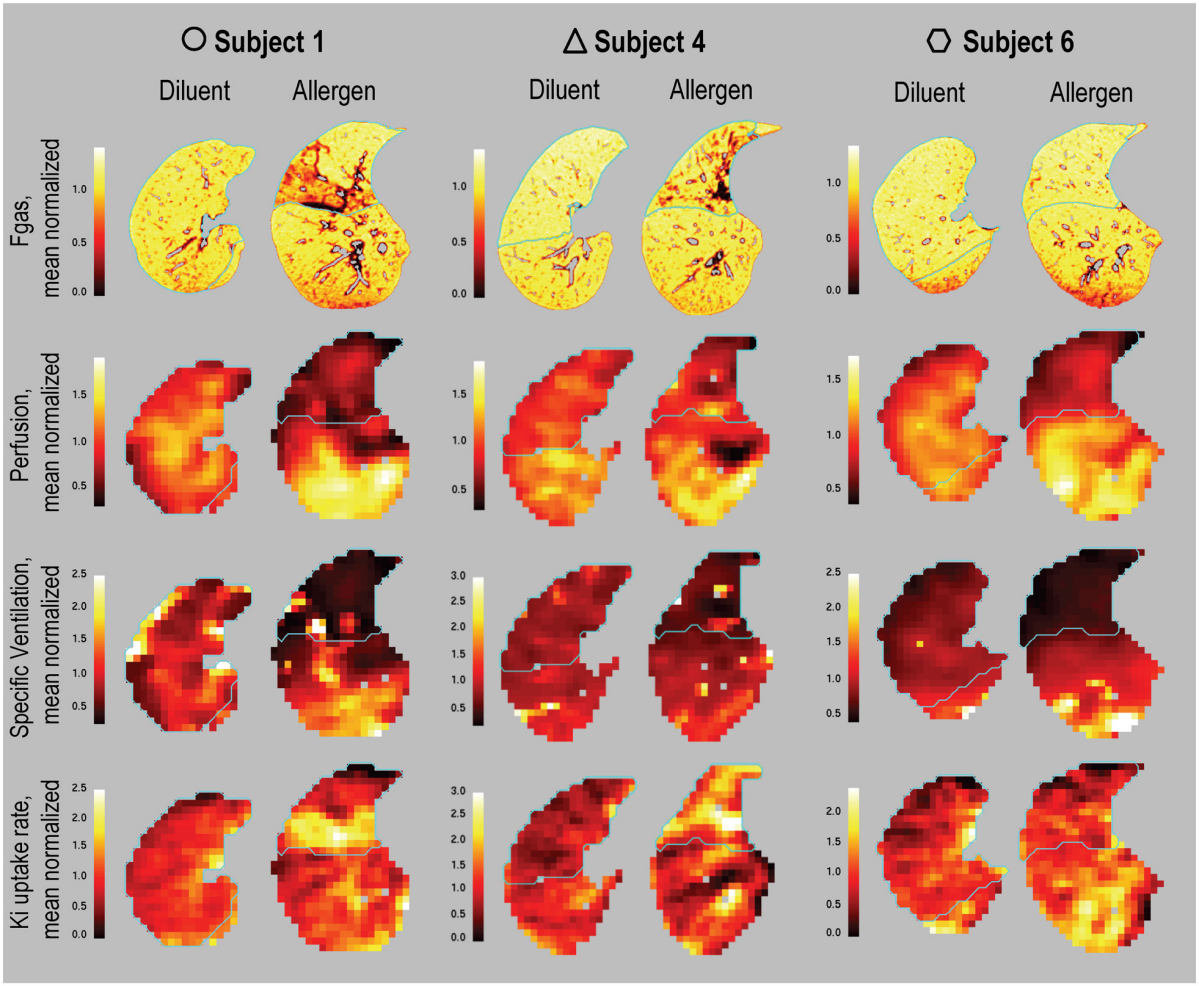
Example images. Representative images of Fgas, Q, sV, and Ki in the diluent and allergen lobes from two subjects who had ground glass opacities present on inspection of HRCT (Subject 1 and 4) and a separate subject with no ground glass opacities (Subject 6). Ground glass opacities are seen as regions with increased red and black colors. In each image, the boundaries of the diluent and allergen lobes are outlined in blue. Note: Color scales differ between subjects. Q, sV and Ki are represented as relative values, relative to the mean of the imaged lung.
